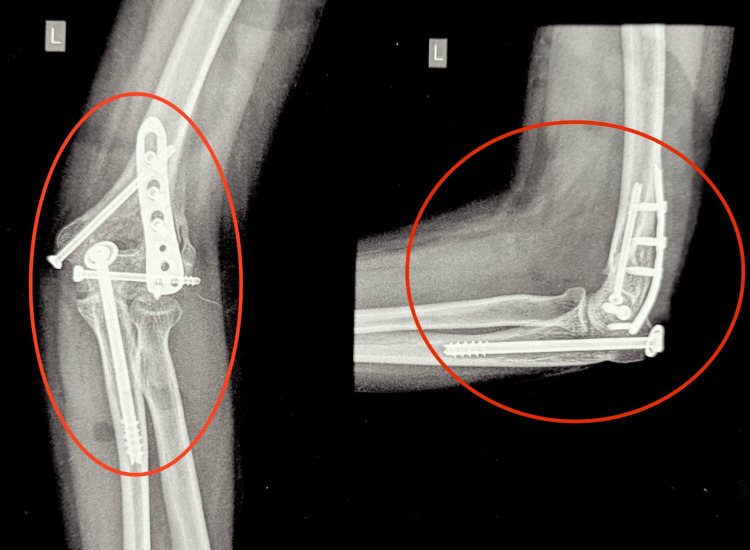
Anteroposterior and lateral radiographs of the left elbow on presentation after primary surgery with implants in situ
